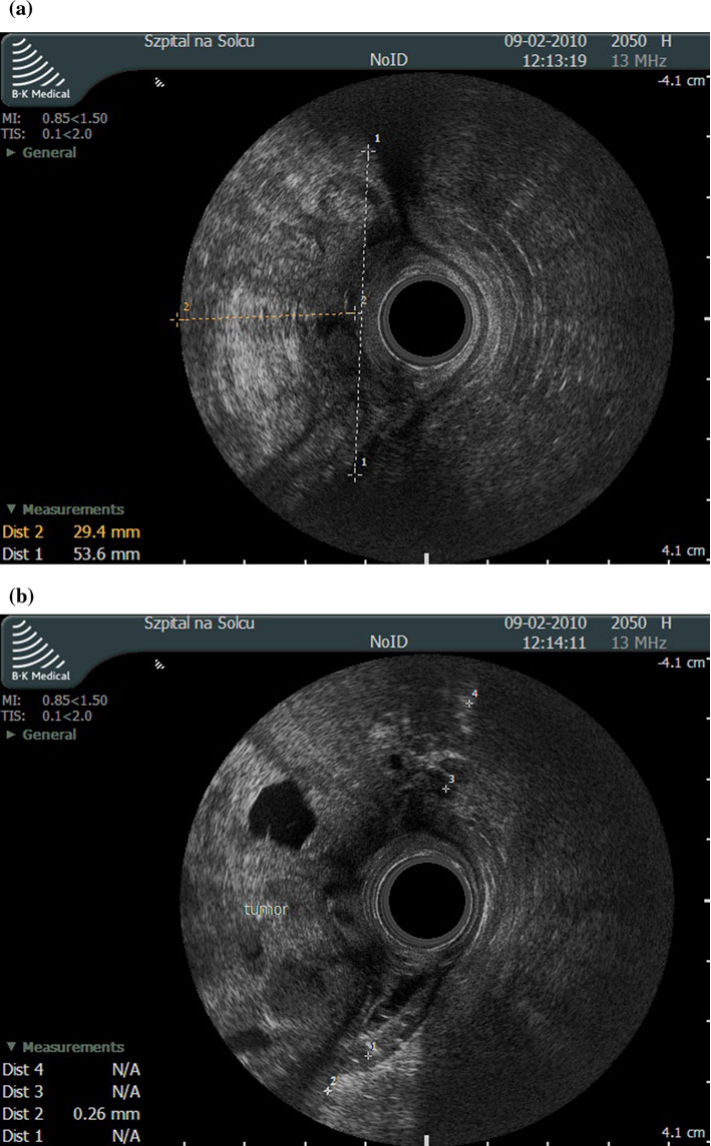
a The endosonographic study shows a hyperechoic tumor, measuring 30 × 53 mm in a transversal plane, taking up the* right half* of the anal canal lumen, infiltrating the anal sphincters, b With anechoic areas of necrosis
